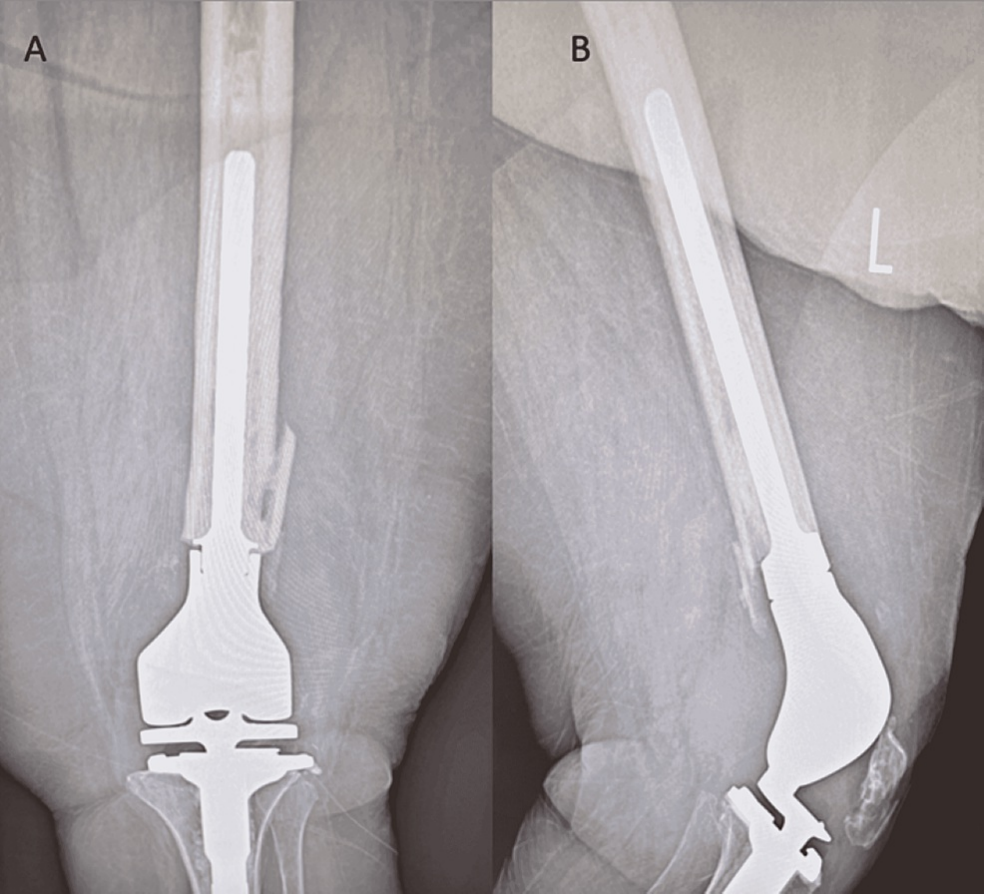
Post-revision surgery imaging demonstrating distal femoral replacement. A: AP X-ray view of the left knee. B: Lateral view X-ray of the left knee revealing patella infera.
